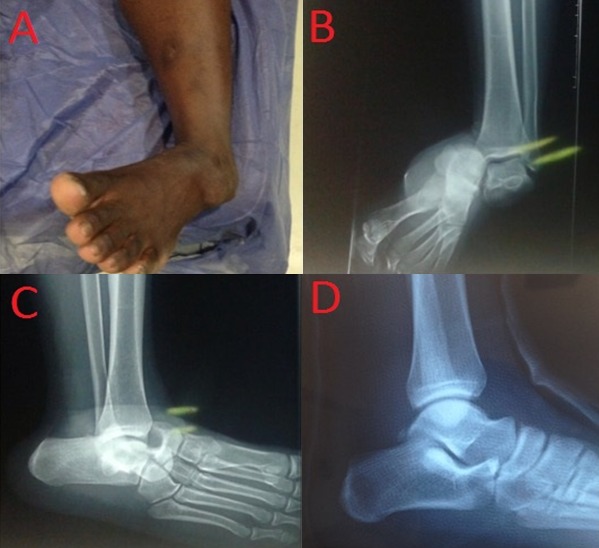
(A) Clinical appearance of a medial subtalar dislocation of the foot; (B, C) radiographs of the left ankle demonstrating a medial subtalar dislocation; (D) radiographs after reduction and immobilization with a posterior splint
